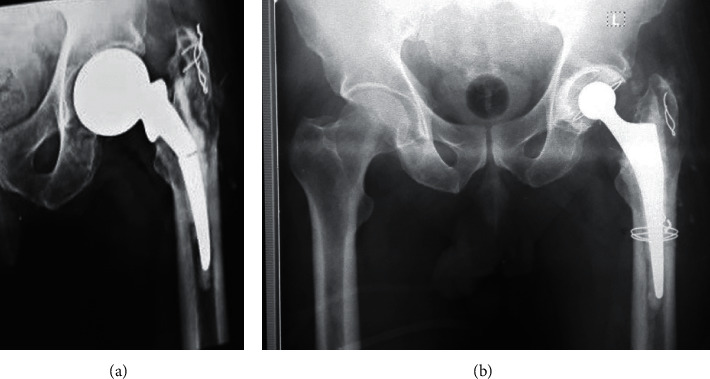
Preoperative and postoperative plane X-ray of a case with broken Thompson prosthesis. Removal of the broken stem by lateral bone window and revision by cemented polyethylene cup and cemented stem.
